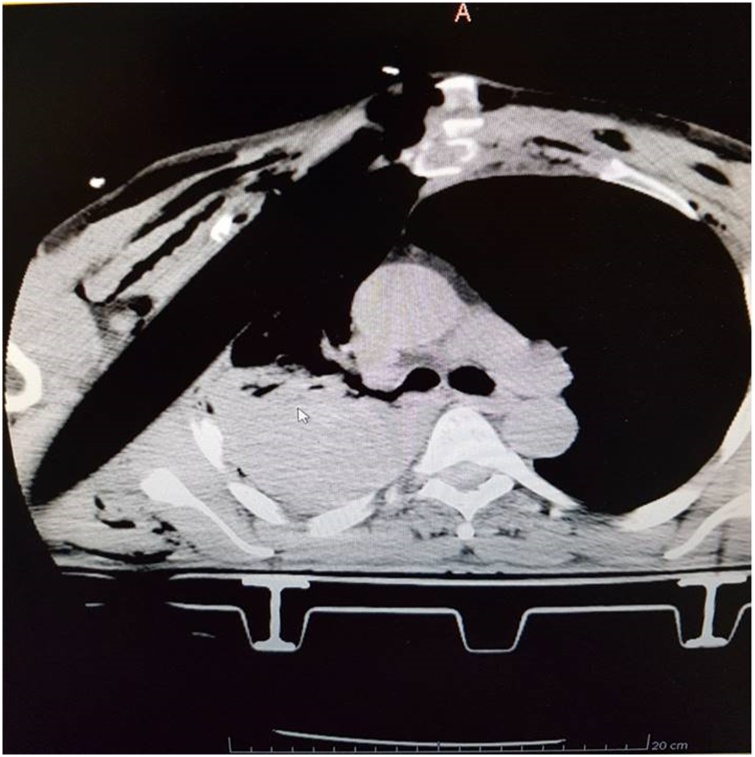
Chest CT scan: Tubuliform foreign body in the right lateral thoracic wall. Conspicuous apical-parietal-basal hemothorax with concomitant atelectasis. Front-basal right pneumothorax and decomposed fractal of the middle arch of the 3rd and 4th ribs.
